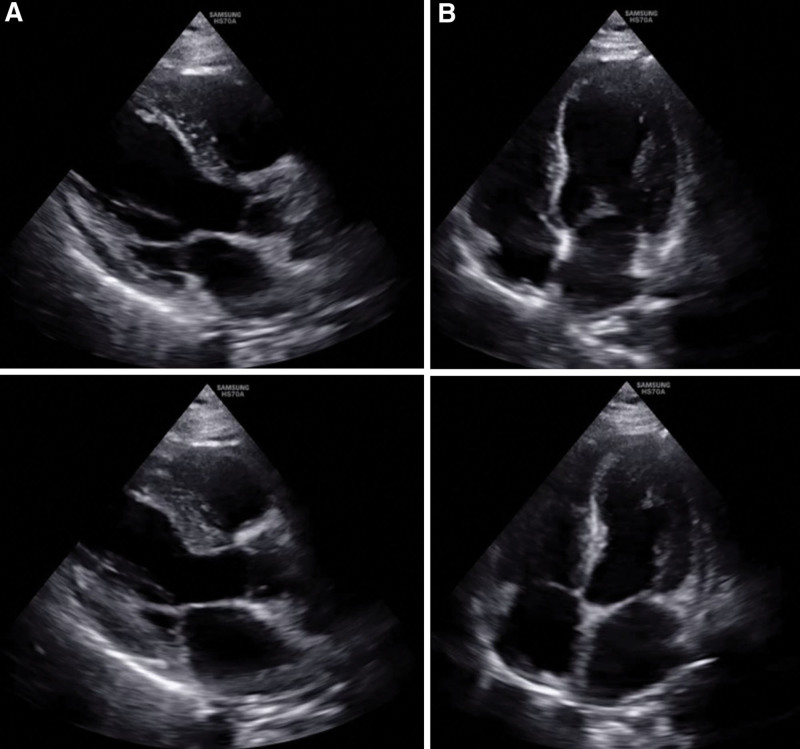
Follow-up POCUS of the end-diastolic and end-systolic phases performed 1 day after admission shows full recovery with a normalized ejection fraction and no regional wall motion abnormalities. (A) Parasternal long-axis view of the end-diastolic (upper) and end-systolic (lower) phases. (B) Apical 4-chamber view of the end-diastolic (upper) and end-systolic (lower) phases.
